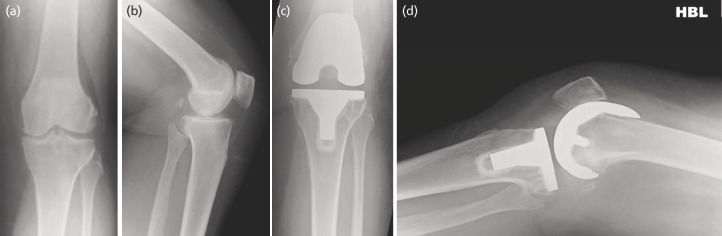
Patient 1, (a) pre-operative antero-posterior radiograph, (b) pre-operative lateral radiograph, (c) post-operative antero-posterior radiograph at one-year follow-up; showing stable isolated tibial lucency in zone 4, and (d) post-operative lateral radiograph at one-year follow-up.
